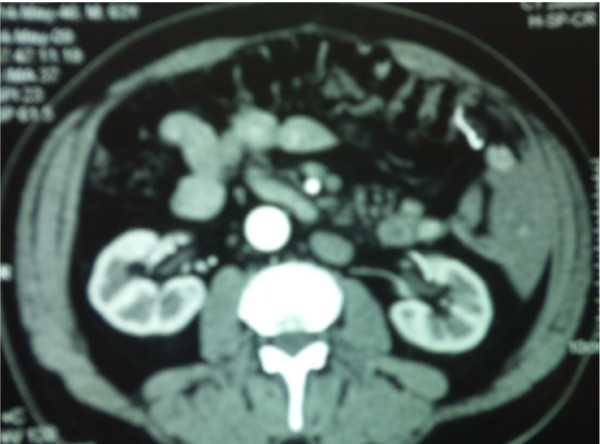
Representative CT images of the abdomen one year after the operation during the patient's follow up. The situs inversus totalis is clearly demonstrated.
